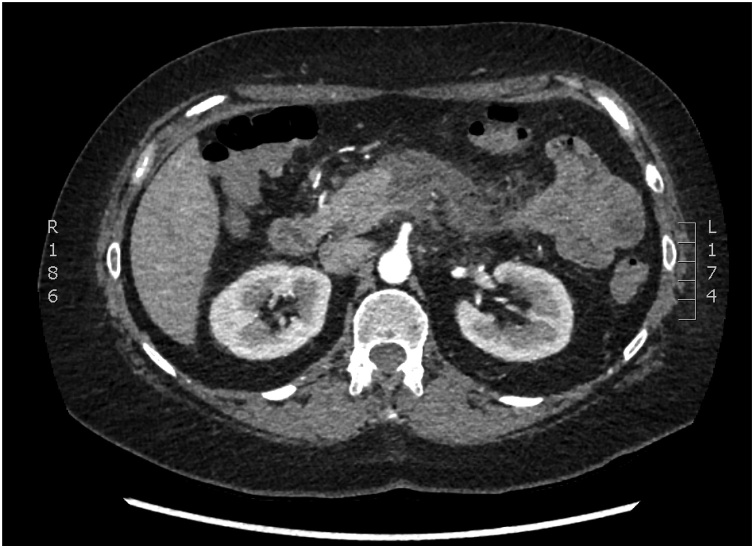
CT Abdomen revealing marked inflammatory stranding around the body of the pancreas, consistent with pancreatitis.
